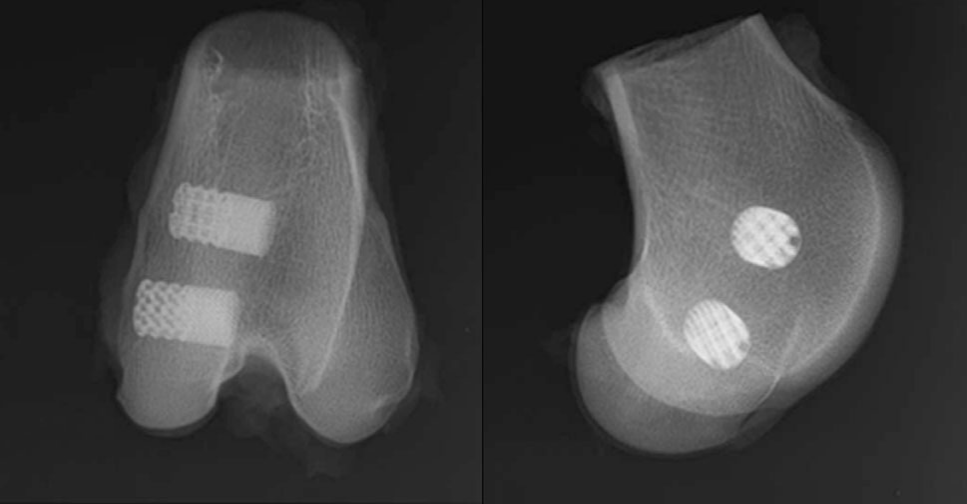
Radiograph showing implant and defect positions within the femoral condyle.
